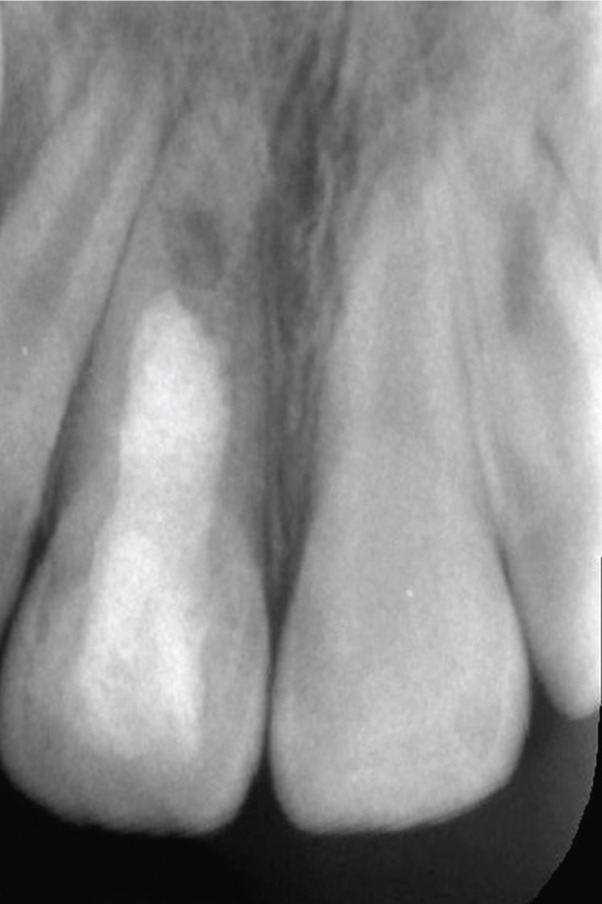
Follow-up at 15 months. Periapical lesion diminished. Maturation of root apex and increase in root canal wall thickness are apparent.
